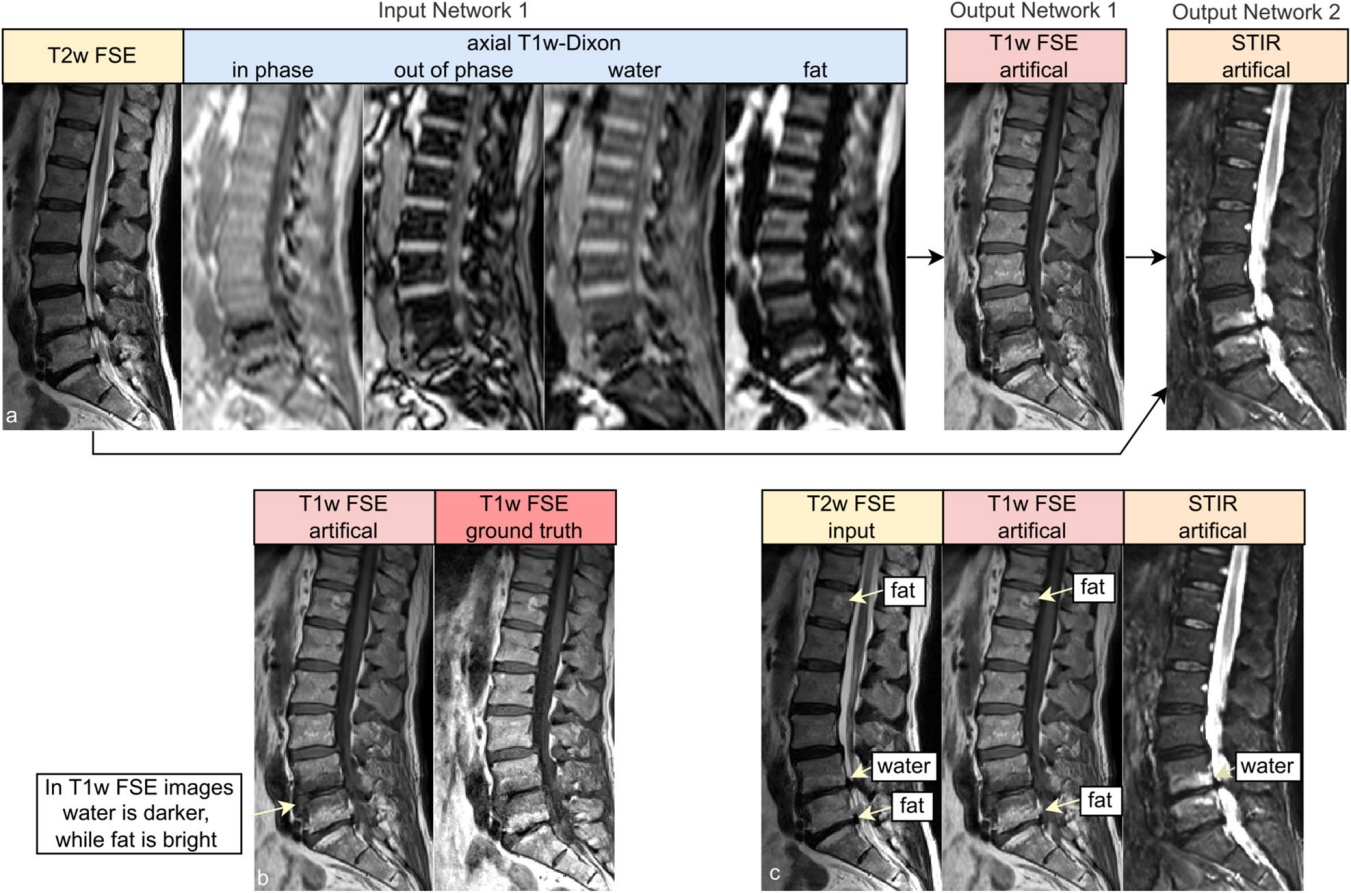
Sagittal views of input and output data. The image is from a participant of the “Study of Health in Pomerania” with Modic changes in L4/5 and L5/S1. a Example input and output of networks 1 and 2. The last vertebra contains a high-water content adjacent to L5/S1 (Modic 2), while it has high fat adjacent to L4/5 (Modic 1). The high-fat content can be seen in the T1w-Dixon fat image, while the high-water content is more difficult to distinguish. Network 2 receives the original T2w FSE image and the generated T1w FSE output of network 1. b A comparison of the generated and real image. The bone structures, the fat, and water aggregation are generated correctly. Only the stomach area differs because time has passed between the real T1w and T2w FSE image due to breathing, the digestive process, and filling their bladder during the examination. c Comparison of expression of water and fat in T1w, T2w FSE, and STIR images. In the STIR, the different hydronation of the inter-vertebra disks is easier to see than in the T2w FSE. STIR, sagittal short tau inversion recovery; T1w-Dixon, axial T1-weighted gradient echo Dixon technique; T1w, sagittal T1-weighted image; T2w, sagittal T2-weighted; FSE, fast spin echo image
